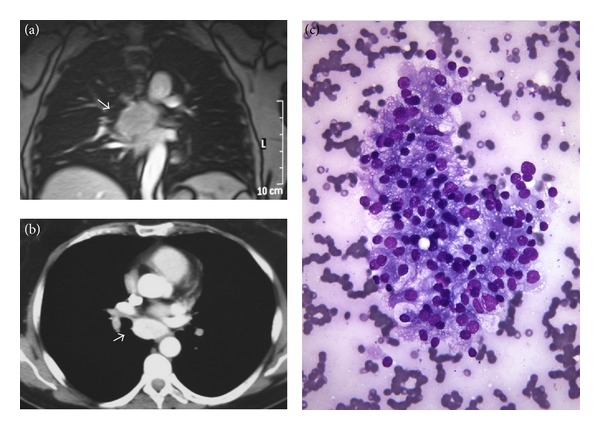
Metastatic renal cell carcinoma. (a and b) Computed tomography showed the renal metastatic mass (arrow) in the mediastinum. (c) Fine-needle aspiration biopsy guided by endoscopic ultrasonography of the mediastinal tumor revealed clusters of epithelial malignant cells morphologically fitting with metastatic clear cell renal cell carcinoma (Diff-Quick).
